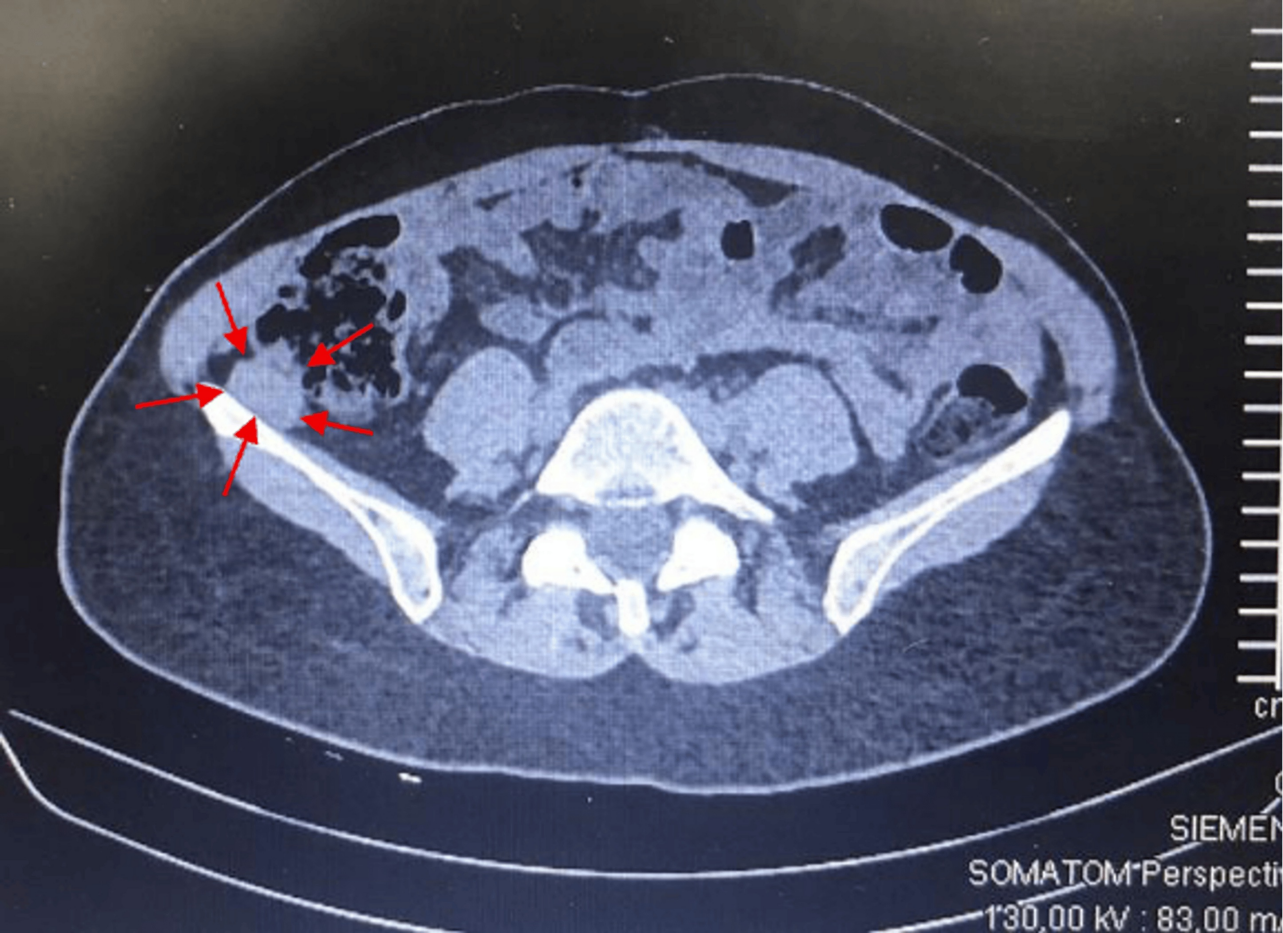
Abdominopelvic CT scan showing a space-occupying soft tissue density lesion in the right iliac fossa, with well-defined borders and contrast enhancement, measuring 27 × 14 mm (outlined with red arrows).
